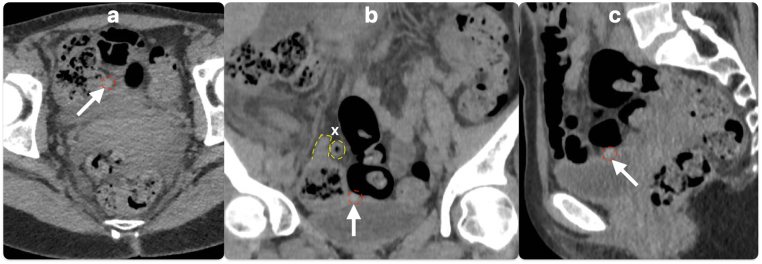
CT images showing axial (a), coronal (b) and sagittal (c) views of the duplicate appendix which was not identified initially. The white arrows indicate the duplicate appendix (outlined in red); the inflamed retrocaecal appendix (x) can also be seen lying superior to the duplicate appendix in panel b (outlined in yellow).
